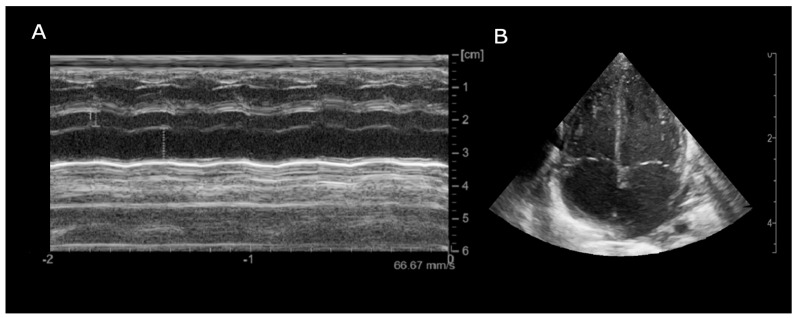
Echocardiogram (month +8), (A): Parasternal long-axis M-mode (LA/Ao ratio 2.18); (B): Apical 4-chamber B-mode showing bilateral atrial enlargement.
